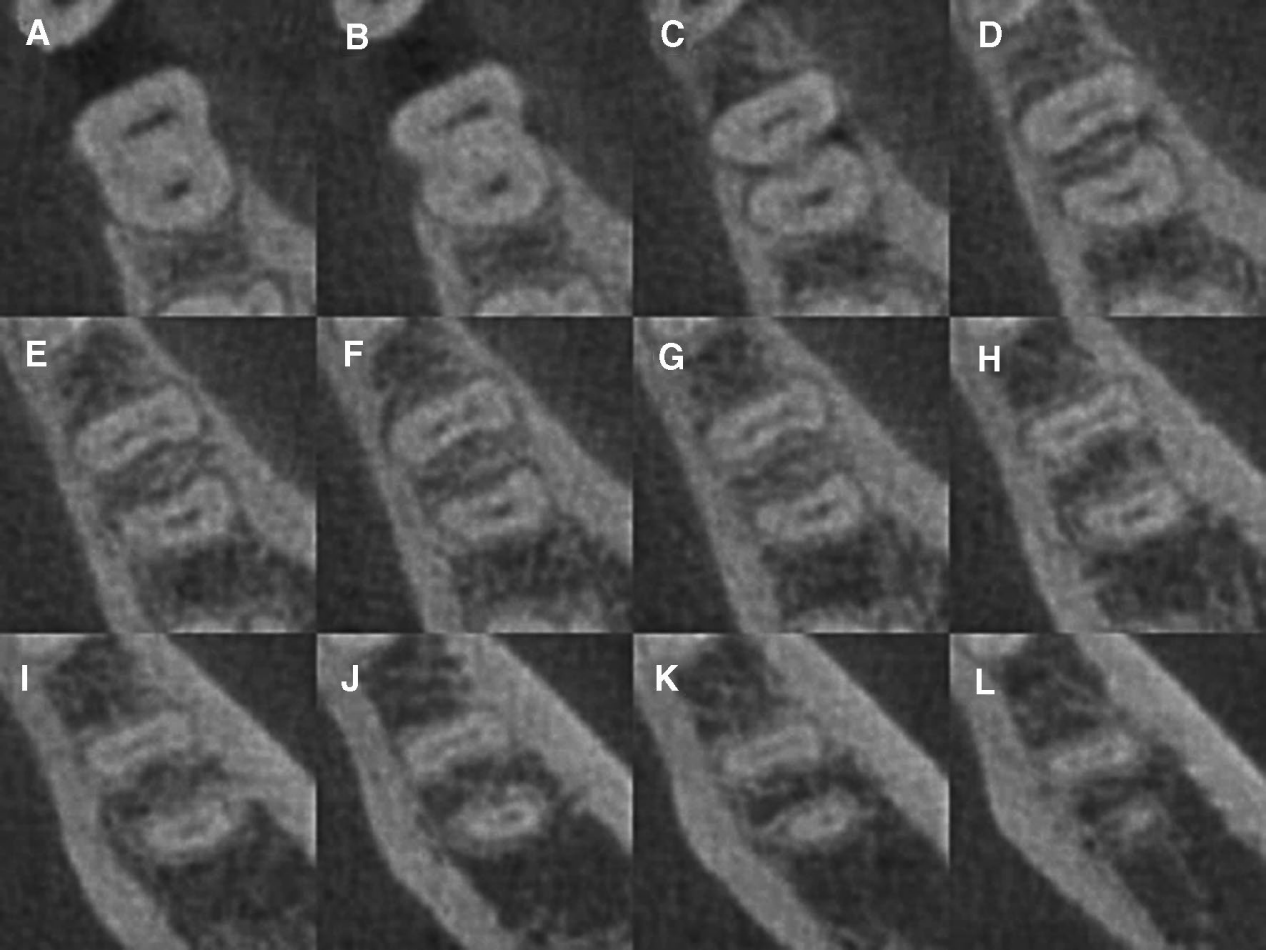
CBCT images showing the isthmus at different axial view levels of the mandibular first molar (tooth #30, Weine type III configuration) (coronal, middle, and apical). a–d Axial view of the mandibular first molar, reoriented to the long axis of the tooth to demonstrate the presence of isthmus, cervical section, e–h middle section, and i–l apical section
